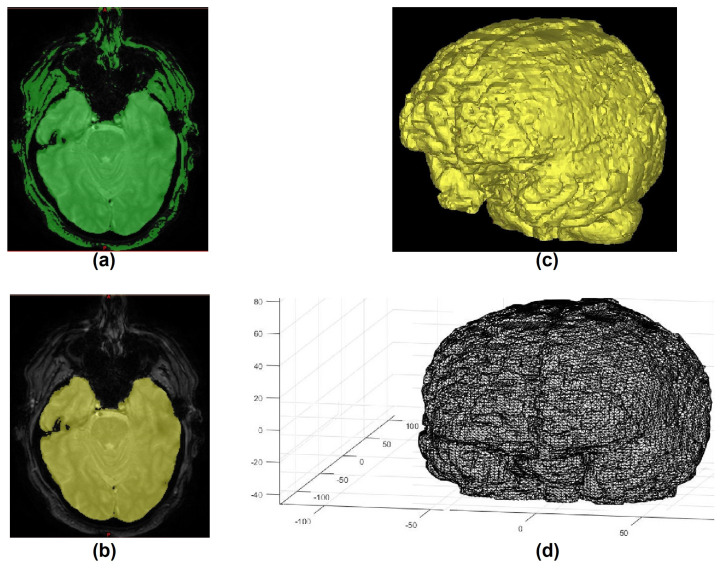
(a) thresholding applied in MRI, (b) region growing, (c) 3D mesh model (STL model), and (d) STL model converted into matrix.
